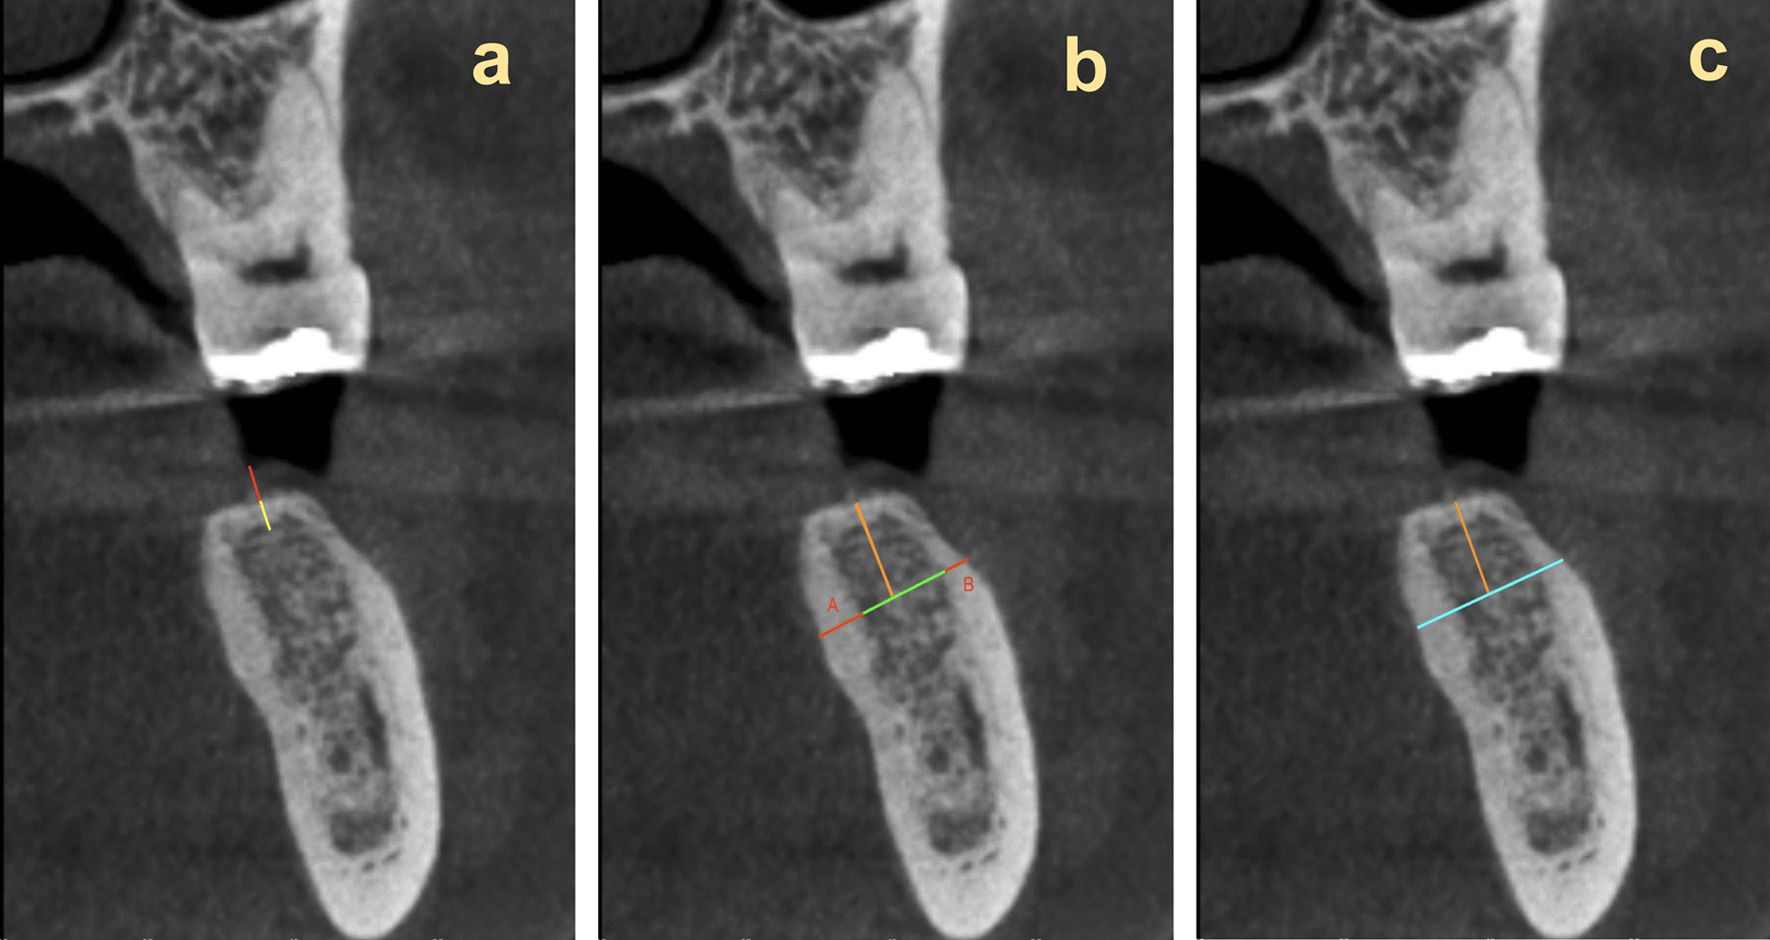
Measurements evaluated on CBCT images a Crestal soft tissue thickness measured in red and cortical bone of alveolar crest measured in yellow. b 5 mm apical to alveolar crest shown in orange. Lingual cortical plate (A) and buccal cortical plate (B) thickness measured in red. c 5 mm apical to alveolar crest shown in orange. Alveolar ridge thickness measured in blue (including thickness of buccal and lingual cortical plates)
